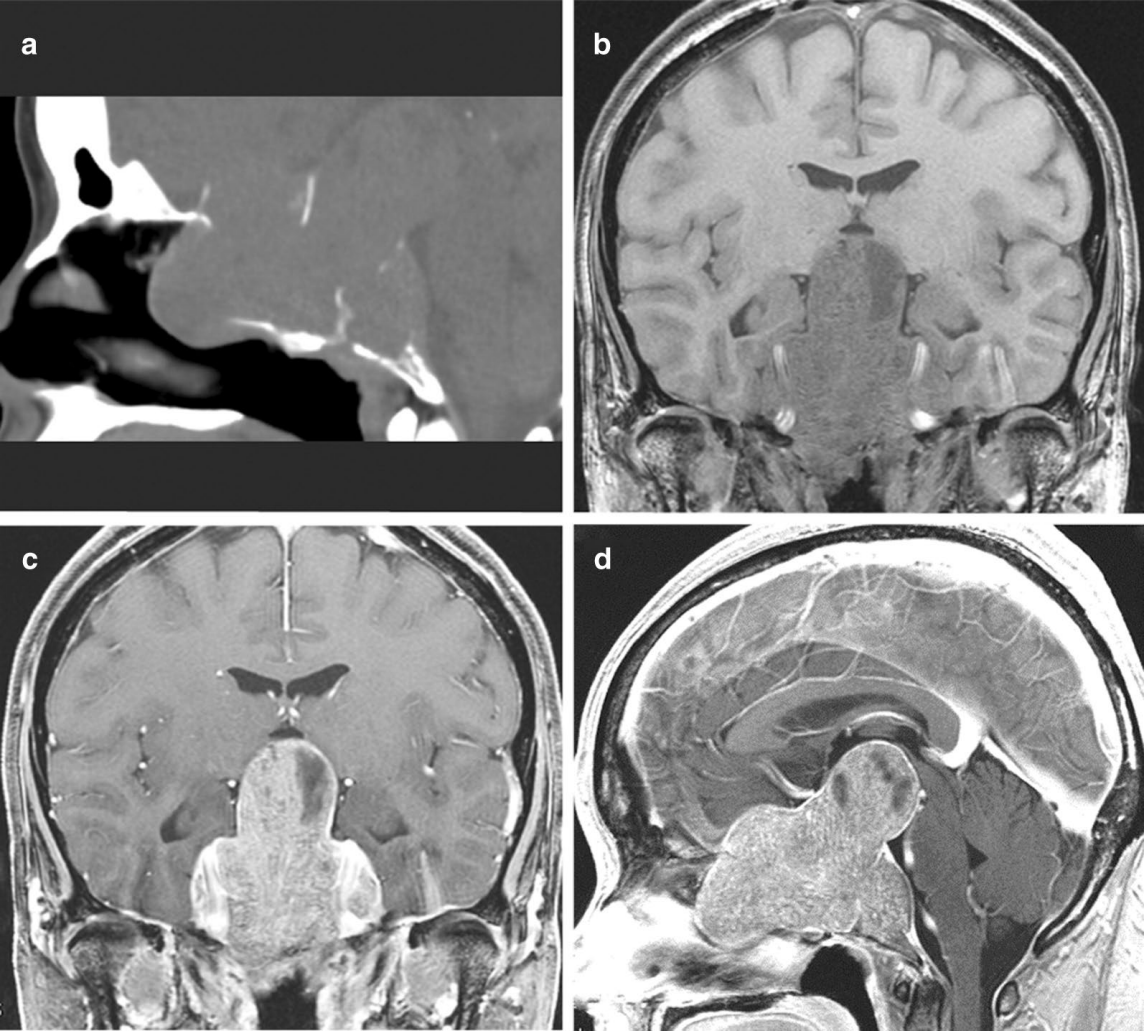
a Sagittal bone computed tomography image showing extensive destruction of the sella turcica. b Coronal T1-weighted magnetic resonance image showing hypointense giant pituitary tumor. c, d Coronal (c) and sagittal (d) contrast-enhanced T1-weighted magnetic resonance images showing marked enhancement of the tumor
